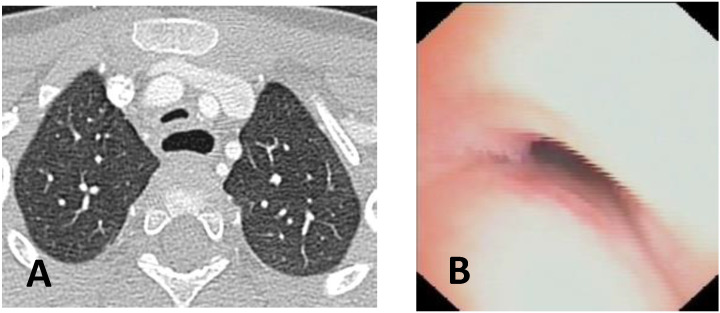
(A) Chest CT with CM: the IA appears well pulled anteriorly towards the sternum, but a severe TM persists with >75% occlusion of tracheal lumen, also related to the presence of a large esophagus compressing the tracheal lumen from behind. (B) The SVBS confirms the persistence of TM, with a patent but severely deformed tracheal lumen even in quit breathing. Abbreviations. CT: Computed Tomography; CM: Contrast Medium; IA: Innominate Artery; TM: Tracheomalacia; SVBS: Static Videobronchoscopy.
